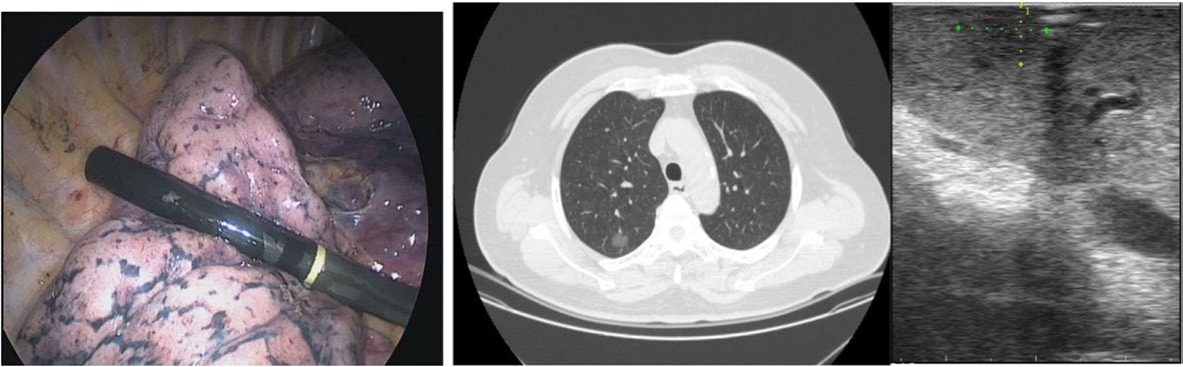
Intraoperative ultrasound localization of pulmonary nodules [54]
